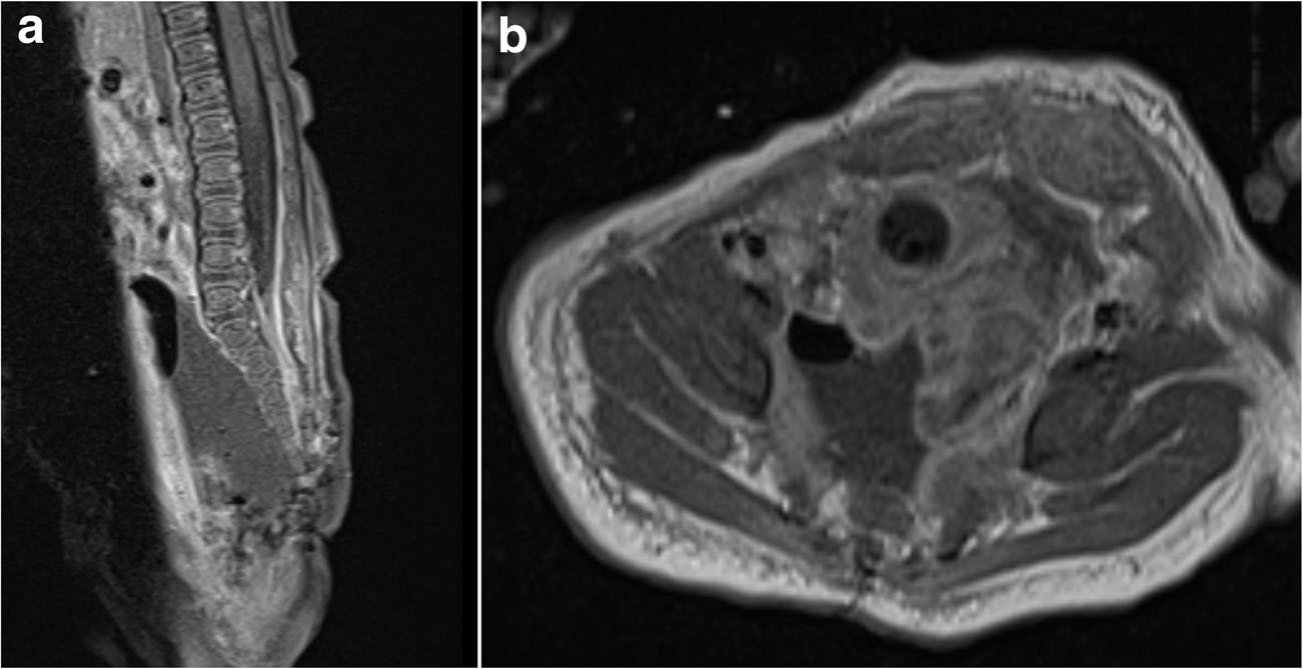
Postoperative, T1-weighted contrast-enhanced sagittal (left), and axial (right) MR images demonstrating a gross total resection without any evidence of residual tumor
